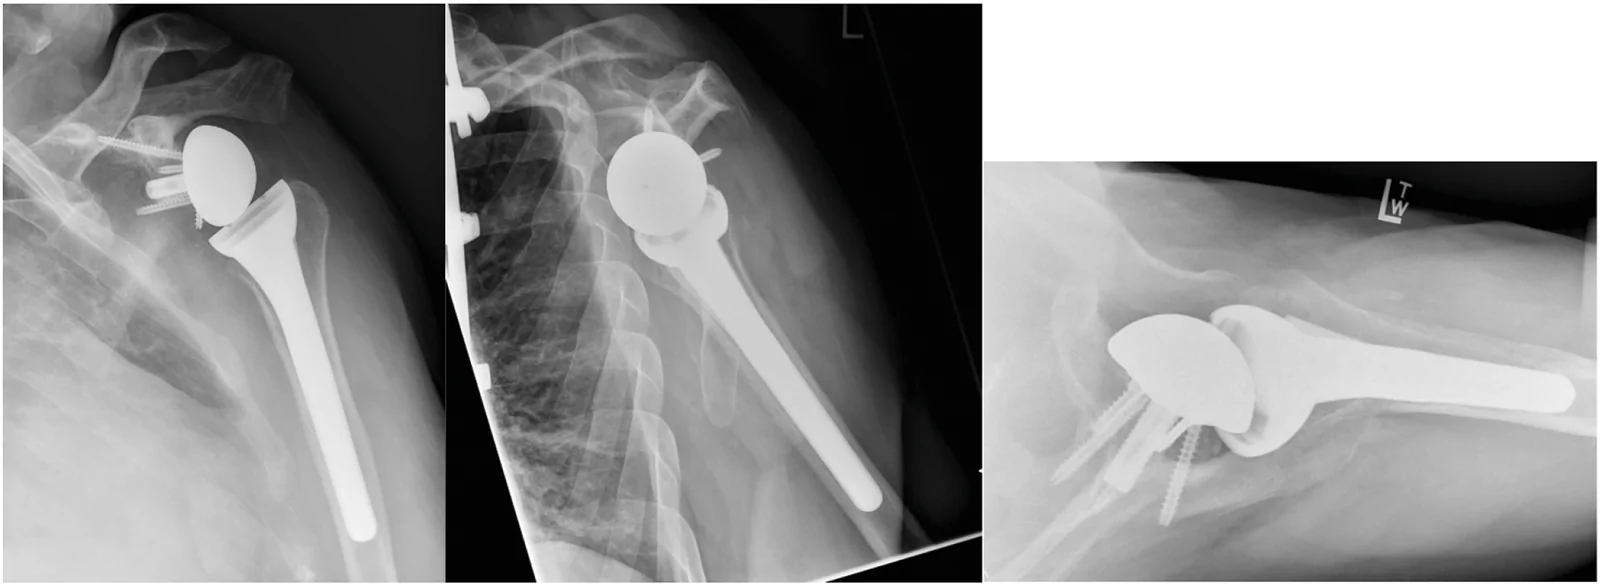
Case 2 post-operative x-rays.
